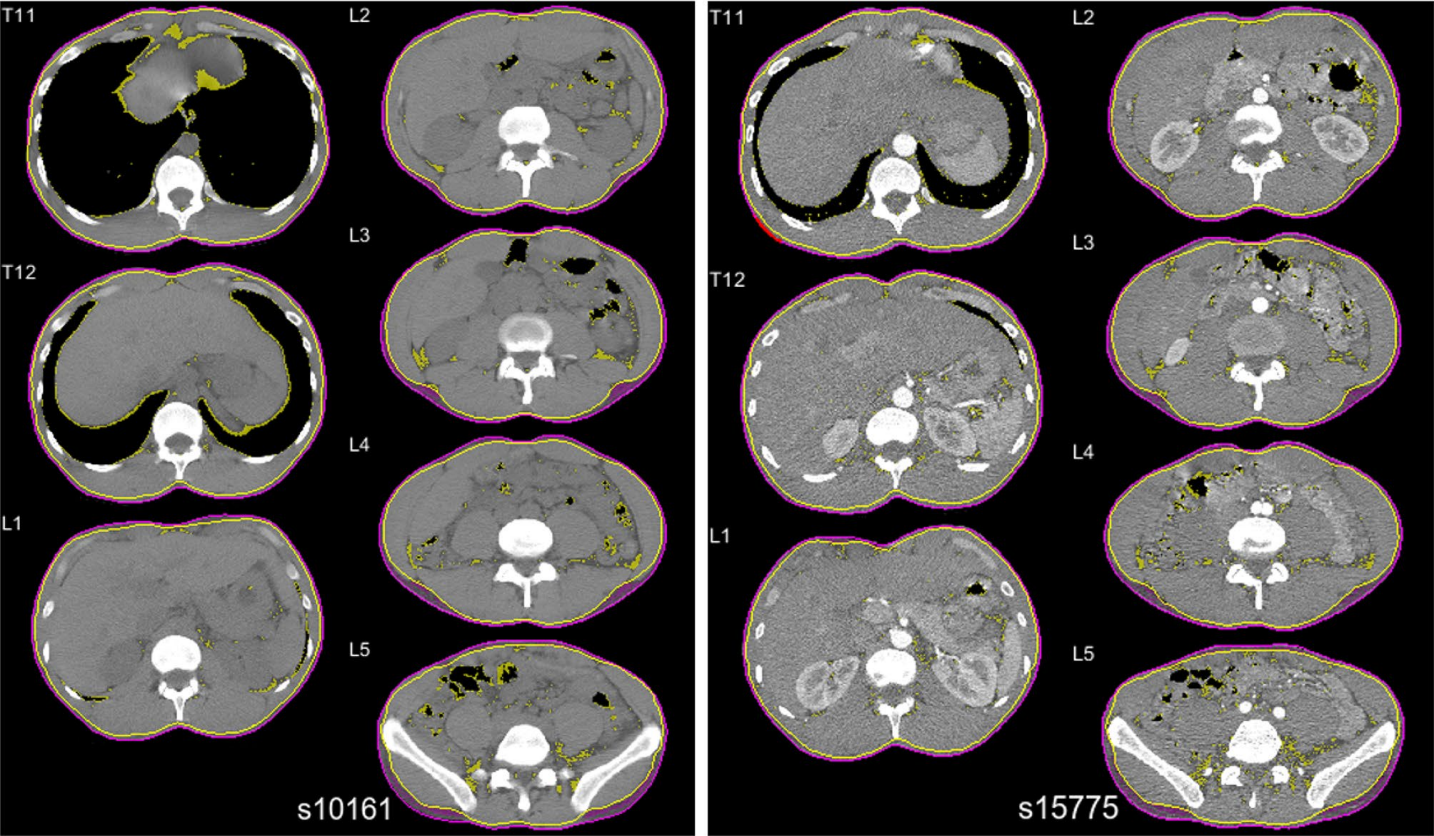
Example of healthy 36 y/o male non-contrast (left) and contrast (right) CT axial slices, showing T11–L5 visceral fat area (yellow-shaded region), subcutaneous fat area (purple shaded region), outer abdominal fascia boundary (yellow line), and skin boundary (purple line). Images demonstrate different lung inflation between contrast and non-contrast scans and resulting shift in organ and fat pixels.
